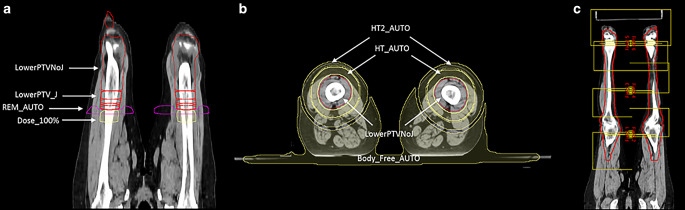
Frontal and transverse views of the main structures generated by the plug-in script on the lower extremities CT (a,b) and the isocenters and fields placed by the script for plan optimization (c). In the text, LowerPTVNoJ and LowerPTV_J are referred to as PTVNoJ and PTV_J, respectively. *LowerPTVNoJ* lower extremities planning target volume (PTV) excluding the junction, *LowerPTV_J* junction structure, *HT_AUTO*, *HT2_AUTO* healthy tissue, *REM_AUTO* remove structure
